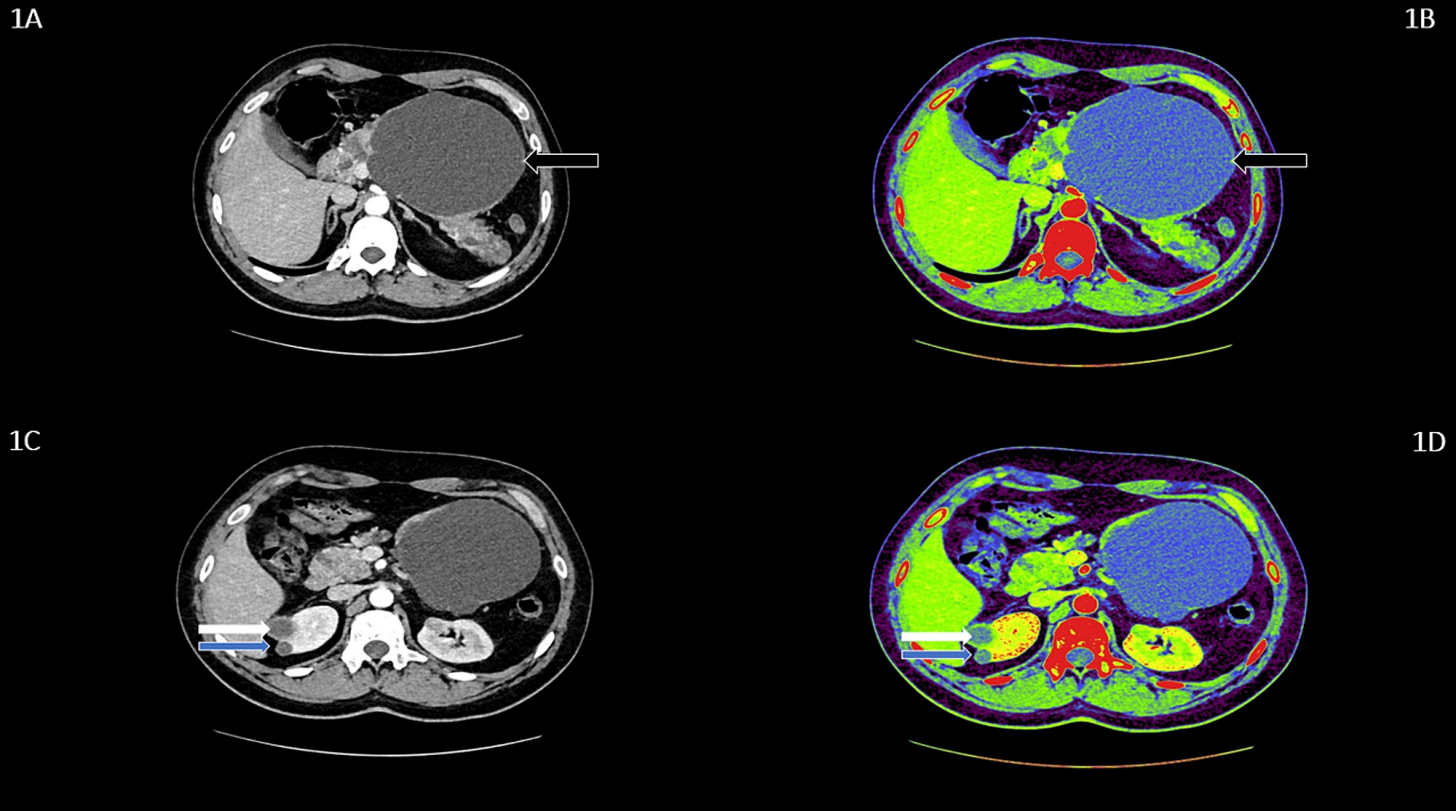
CECT whole abdomen of the symptomatic patient demonstrating pancreatic and renal manifestations 1A and 1B: Axial CECT abdomen shows a pancreatic cystic lesion with few septations, showing the focal calcification in the body of the pancreas (black arrows). 1C and 1D: Axial CECT abdomen shows a well-defined cystic lesion, showing the peripheral enhancement and a peripherally placed enhancing soft tissue attenuation nodule within, at the upper pole of the right kidney, suggesting a neoplastic etiology (white arrows); and a hypodense cystic lesion at the upper pole of the right kidney, suggesting renal cyst (blue arrows). CECT: contrast-enhanced computed tomography
